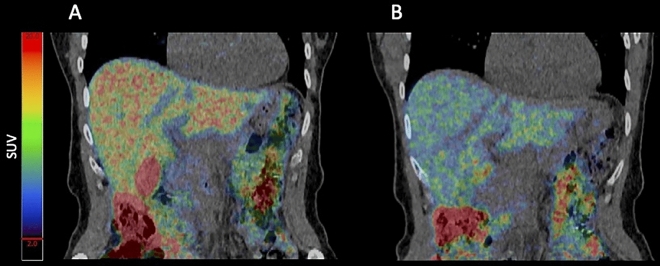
PET/CT scan 13 h after oral intake of 30 MBq copper-64. (A) Baseline scan. (B) End-of-treatment scan—after 4 weeks administration with zinc gluconate 50 mg × 3 daily.
